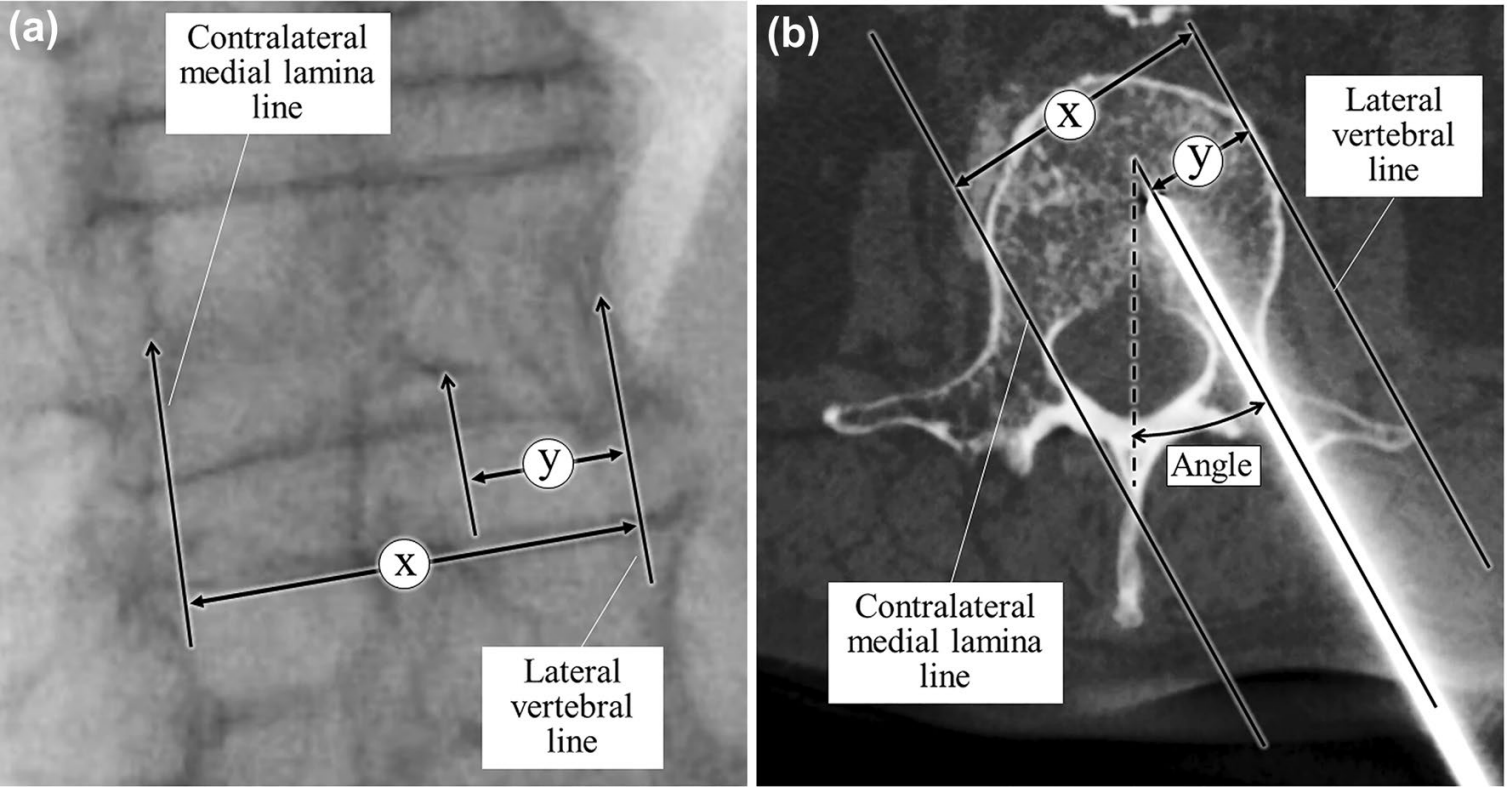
A left anterior oblique fluoroscopic view of the lumbar vertebral body before puncturing (a) and an IVR-CT image of the lumbar vertebral body after the insertion of the bone biopsy needle (b). The right, center, and left vertical arrows in (a) and (b) indicate the lateral medial lamina line, the puncture point, and the contralateral vertebral line, respectively. Vertebral estimated tilting ratio (VET-ratio) is equal to y/x (%), where ‘x’ represents the distance between the lateral vertebral line and the contralateral medial lamina line and ‘y’ represents the distance between the vertebral lateral line and the puncture point shown in (a) and (b). VET-ratio should be 33% in VETERAN method
